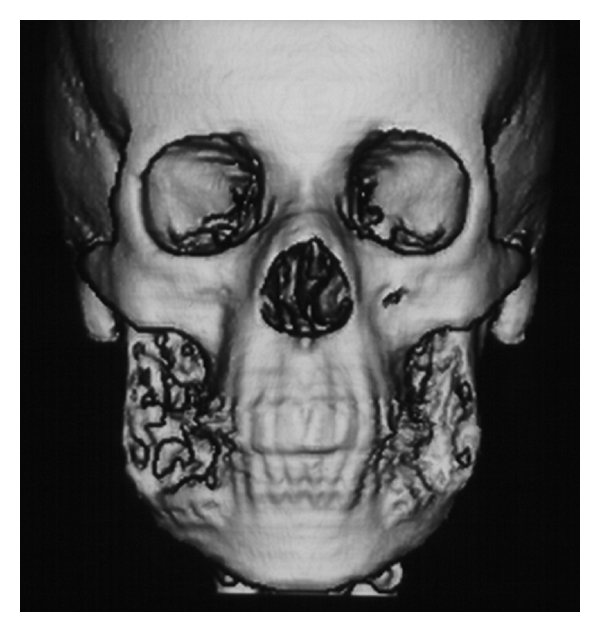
3D reconstruction of the CT scan images showing erosive aspect and multiple depressions at the bilateral vestibular cortical bone of the retromolar region (Gomes et al. [6]).
